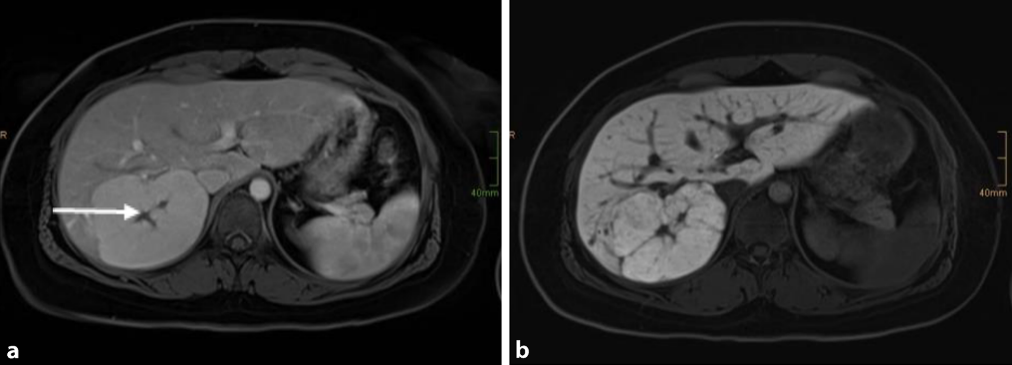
FNH: axial MRI in the late arterial phase (a) with Primovist® (Bayer Schering Pharma, Berlin, Germany) shows a large, slightly hyperintense lesion (*arrow*), with a hypodense centre, in the right liver, consistent with a central scar (*arrowhead*) [9]. The hepatobiliary phase (b) images show a strong uptake of Primovist, which is a typical sign of FNH
